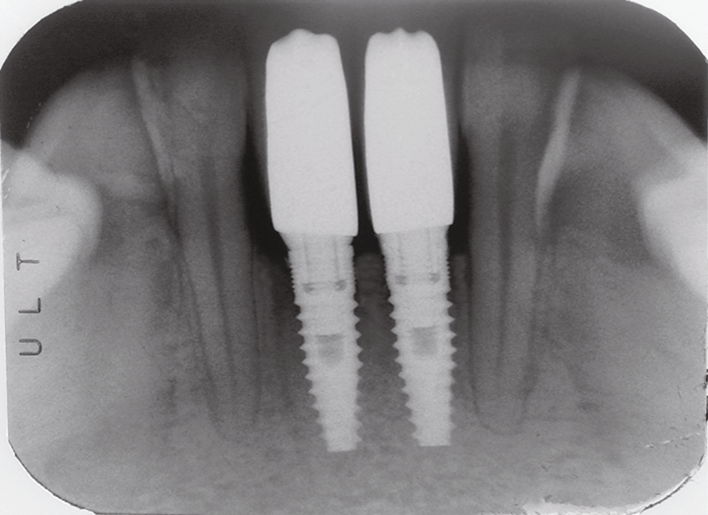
Periapical radiograph taken 2 years after prosthetic loading.
